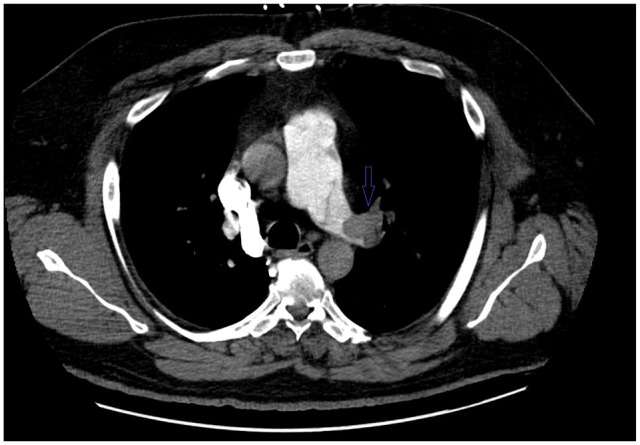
Computed tomography pulmonary embolism (CTPE) showing left pulmonary artery PE.
